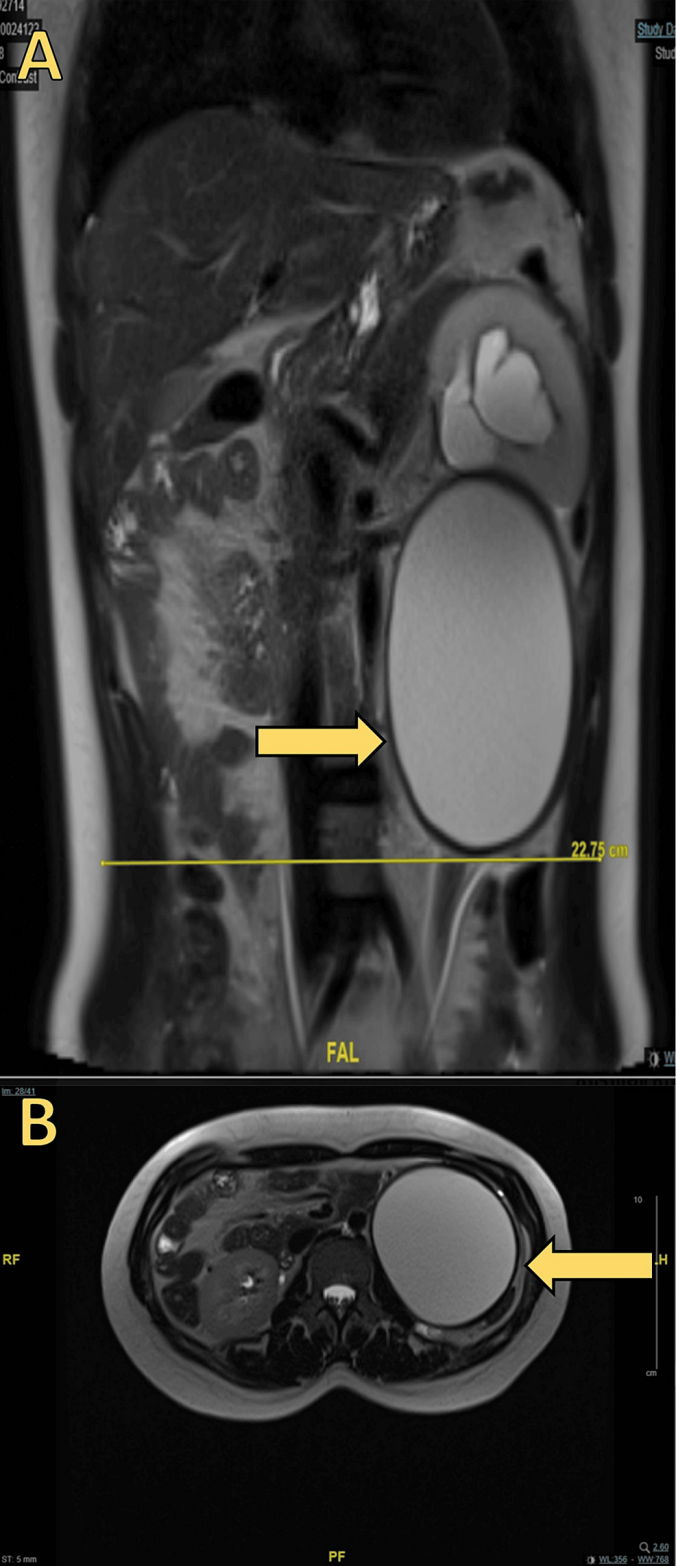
The MRI shows a T2-hypointense, thick-walled cystic retroperitoneal mass containing a small amount of fat at the superior portion and a fat-fluid level. (A) coronal plane of the abdominal MRI; (b) transverse plane of the abdominal MRI
